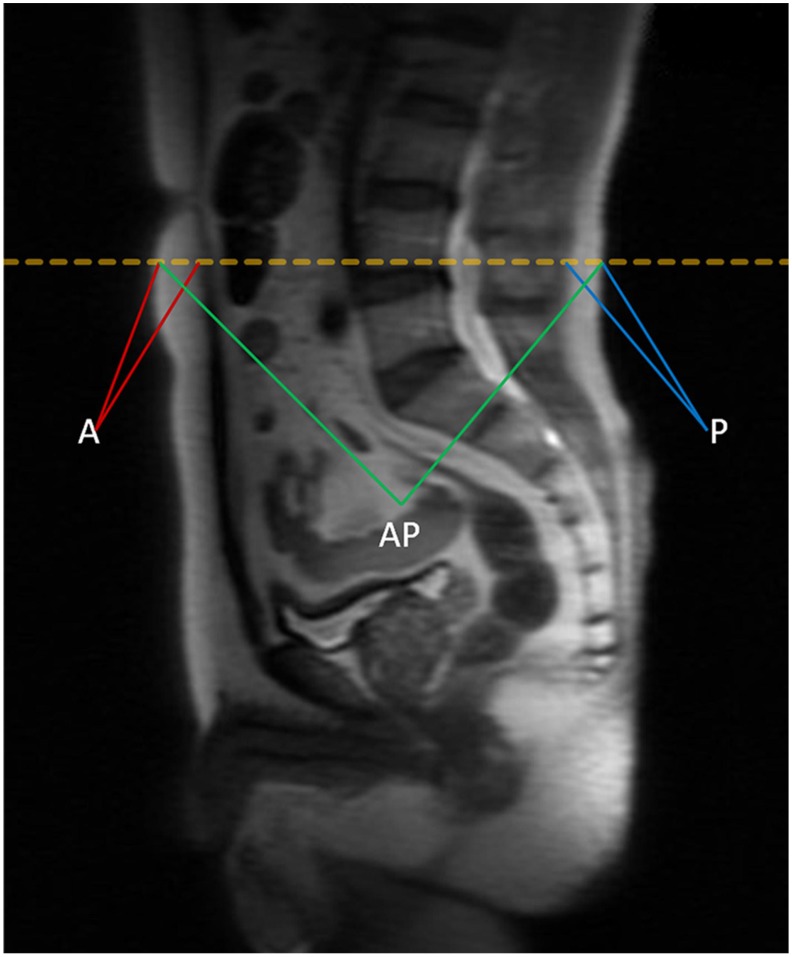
The quantity of anthropometric measures of SAT area was measured by MRI with T2-weighted sagittal localization images. All images were identified at the level of the umbilicus (approximately L4-L5 marked in orange dotted line) for 367 patients in supine position. A in maroon or P in blue represents measurement of the anterior or posterior abdominal adipose thickness respectively, and anteroposterior diameter (AP) was measured in green lines. The value of SAT was calculated as the sum of anterior abdominal fat and posterior abdominal fat (SAT=A+P). The visceral obesity percentage was defined as VAT%, which was calculated using the formula VAT%=[(AP-SAT)/AP]×100%.
